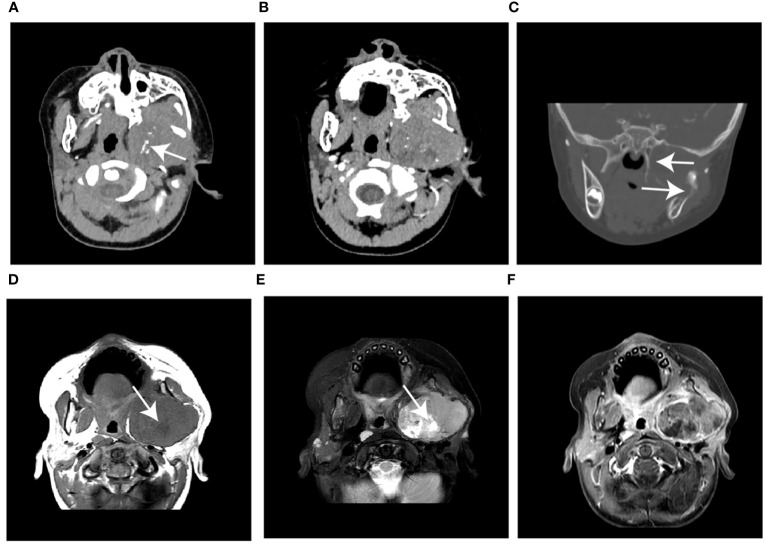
Case 1, a 21-month-old boy with RMS. (A) The unenhanced CT image shows a huge, slightly hypodense mass in the left infratemporal fossa with speckled calcification (arrow). (B) The mass shows heterogeneous enhancement without encasing vessel signs on contrast-enhanced CT. (C) The coronal reconstructed CT image shows extensive erosion of adjacent bones (arrow). (D) Axial T1WI and (E) T2WI show the mass with heterogeneous signals intensity with cystic lesions (arrow). (F) The gadolinium-enhanced T1WI shows a hyperintense solid mass compared with the adjacent muscle layer. WI, weighted image.
